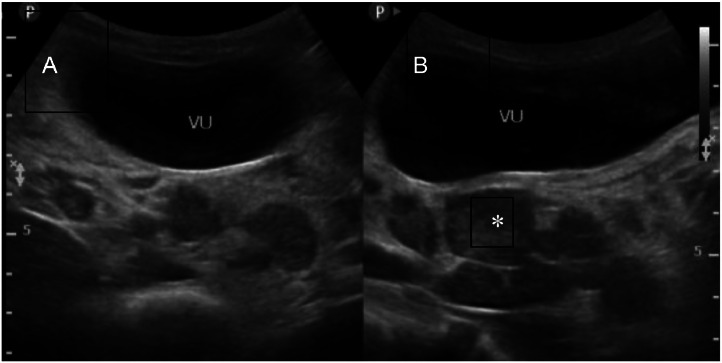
Abdominal ultrasound, transverse (A) and longitudinal (B) sections revealed the presence of multiple well-defined oval hypoechoic masses in the abdominal cavity (*).
